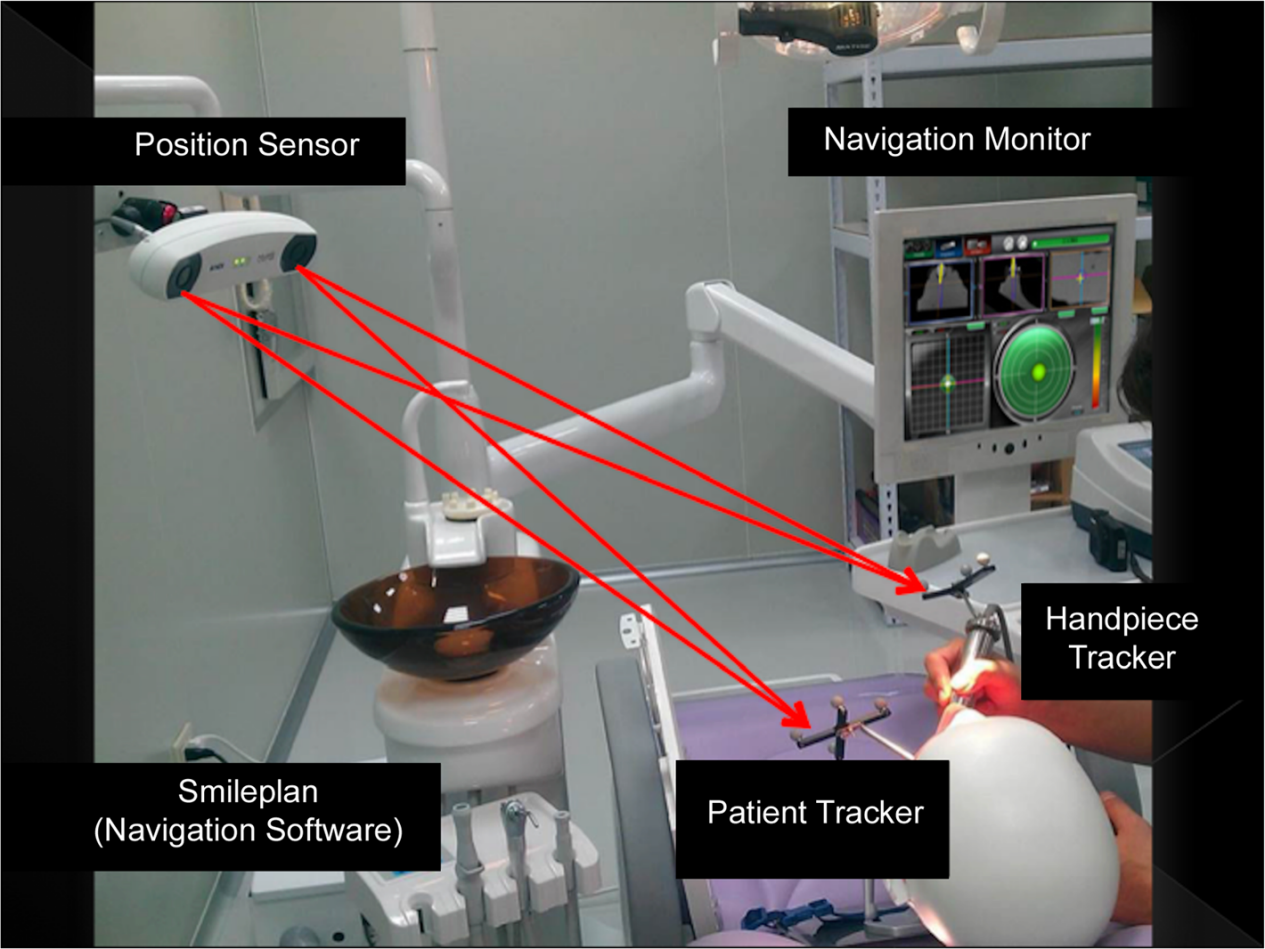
This dental implant navigation system is composed of 3 parts. Cone beam computed tomography (CBCT) and SmilePlan are used to set up the implant treatment plan. A position sensor tracks the handpiece and the patient’s movements in real time. AqNavi monitor guides the surgeon in positioning the handpiece in the optimal location for accurate drilling. (Figure was provided by Taiwan Implant Technology Company, Ltd.)
